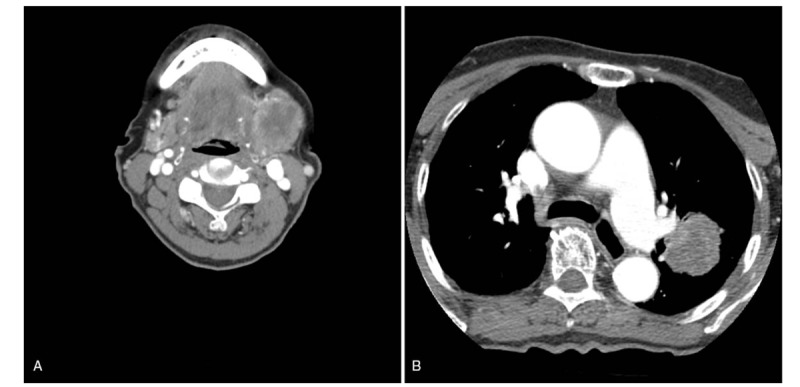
CT scans demonstrate a 3.7 × 3.1 cm sized heterogeneous enhanced, central necrotic lesion with irregular margin in the left submandibular gland (A), and approximately 3.7 × 3.2 cm sized mass in the left upper lobe (B).
